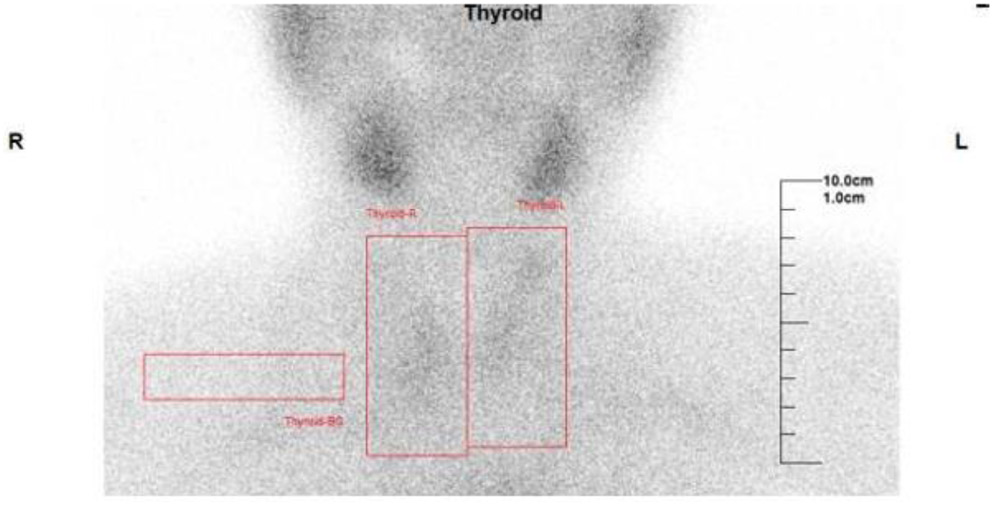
99m-Technetium scintigraphy. 99m-Technetium scintigraphy showed a decreased tracer uptake at 0.4% (reference range: 0.5–4.0%).
